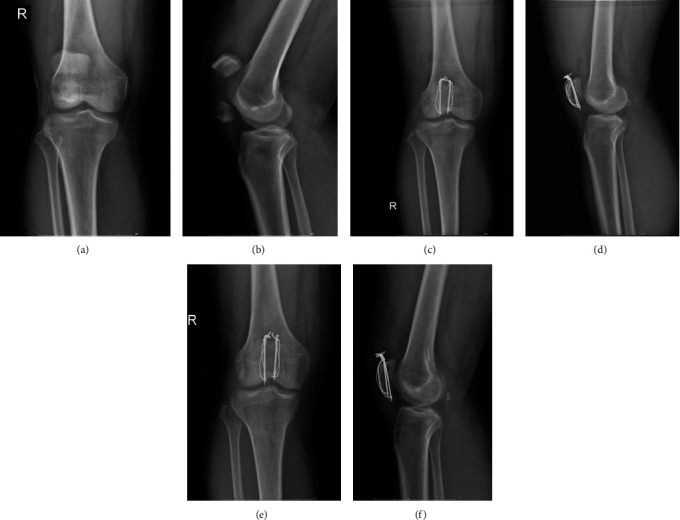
(a–f) A 44-year-old man with a transverse patellar fracture had K-wire tension band fixation. (a) An anteroposterior X-ray shows the transverse patellar fracture after injury. (b) A lateral X-ray after injury. (c) A postoperative anteroposterior X-ray of 1 month. (d) A postoperative lateral X-ray of 1 month. (e) A postoperative anteroposterior X-ray of 6 months. (f) A postoperative lateral X-ray of 6 months.
